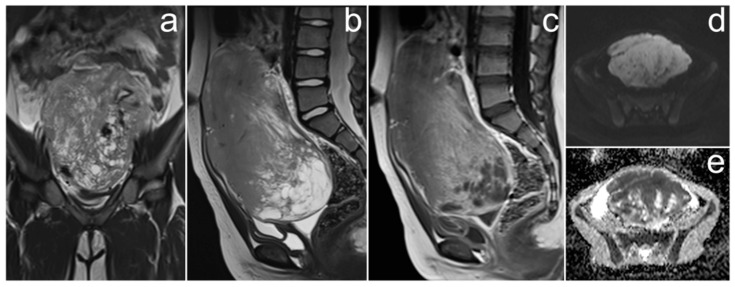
The MRI of primitive neuroectodermal tumor/extraskeletal Ewing sarcoma of the left ovary in a 10-year-old patient (a–e). (a,b)—FSE/T2; (c)—post-contrast FSE/T1; (d)—DWI; (e)—ADC map (images courtesy of Prof. K. Jonczyk-Potoczna, Poznan University of Medical Sciences).
